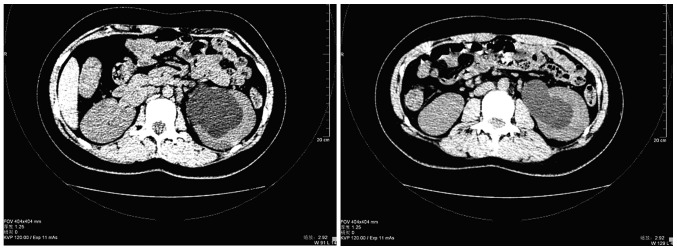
Different computed tomography (CT) scans of the abdomen prior to surgery. This revealed left hydronephrosis and a parapelvic cyst, with no visible stones in the middle and distal segments of the double ureter, and little fluid accumulation in the pelvic cavity.
